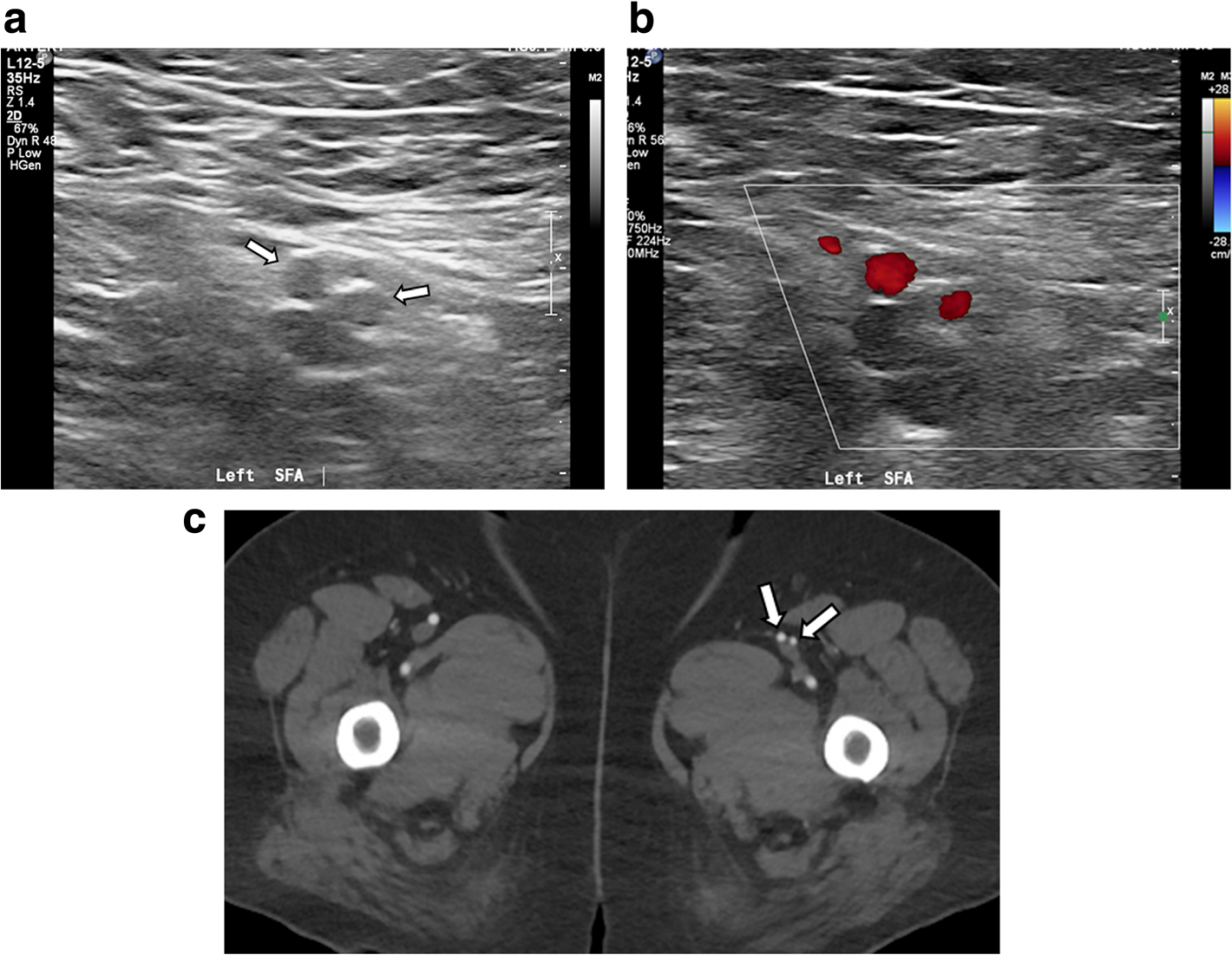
Gray scale (a) and color Doppler ultrasonography (b) show that the left superficial femoral artery is divided into two trunks (arrows) with similar luminal diameter and courses parallel. The axial image of arterial phase CTA of bilateral lower extremity (c) shows the duplicated left superficial femoral artery (arrows). The luminal diameter of the medial one is slightly larger than that of the lateral one. Note normal right superficial femoral artery
